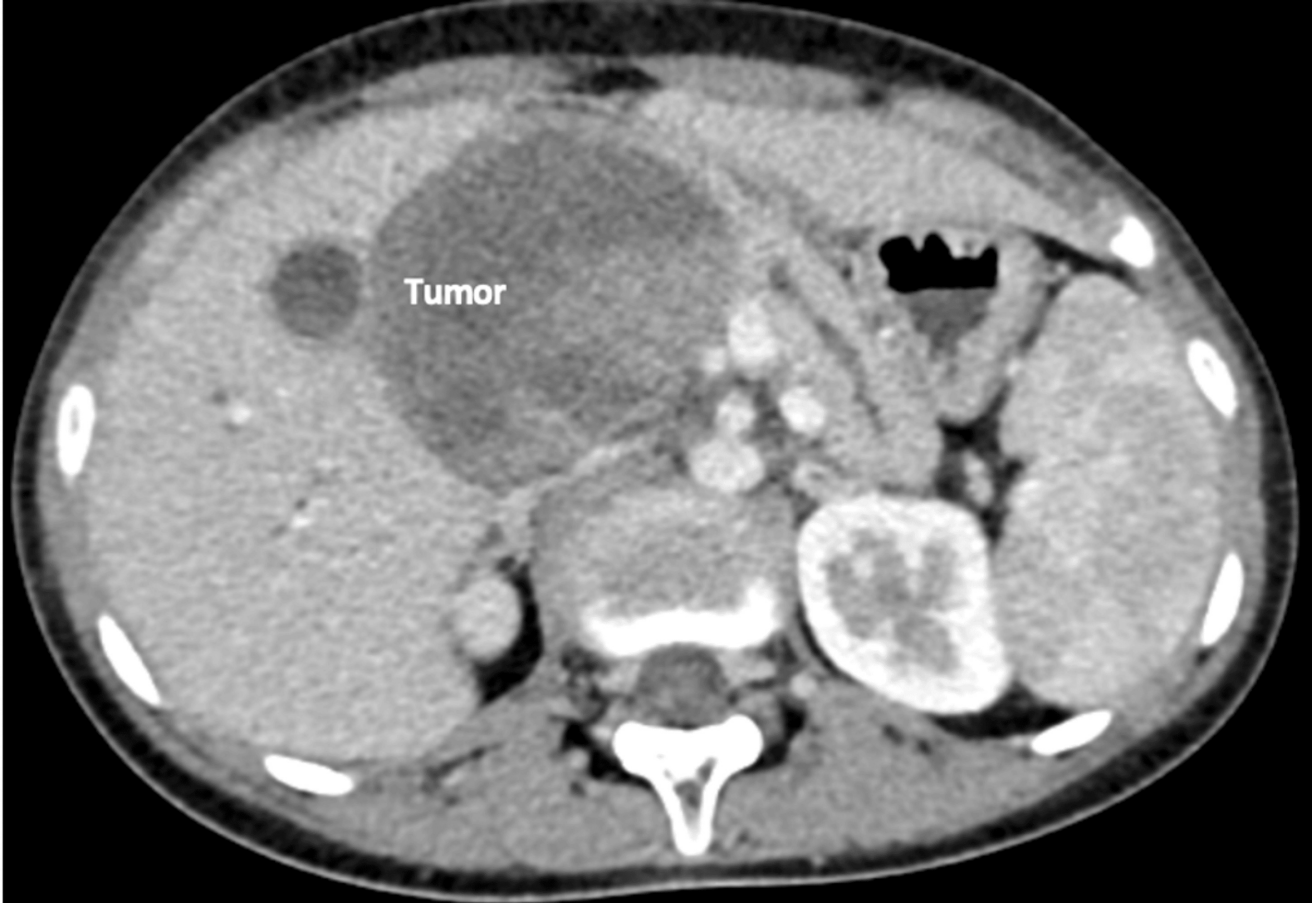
CECT abdomen (axial cut) The tumor labeled is seen to have both solid and cystic areas within it, a pathognomonic feature of SPEN SPEN, solid pseudopapillary epithelial neoplasm; CECT, contrast-enhanced CT of the abdomen
